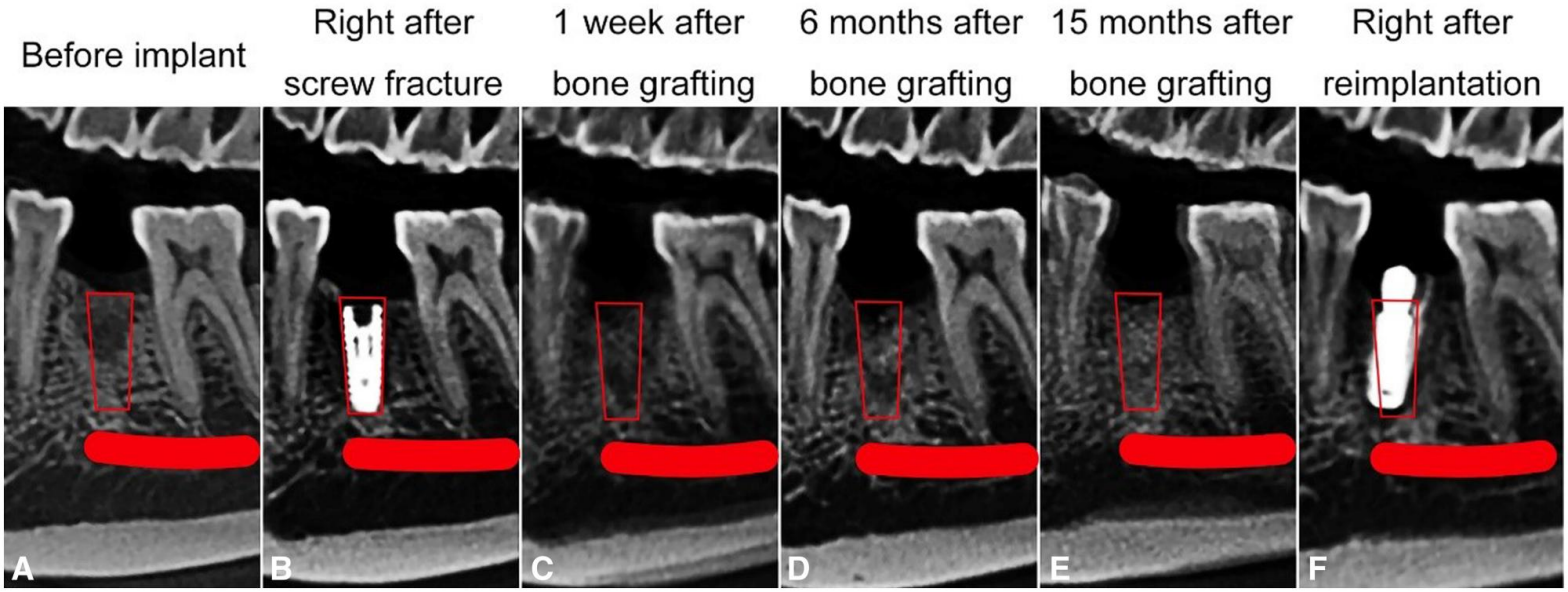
Sagittal CBCT sections at different time points: (A) before implant, (B) immediately after screw fracture, (C) 1 week after bone grafting, (D) 6 months after bone grafting, (E) 15 months after bone grafting and (F) immediately after reimplantation. The red box represents the bone defect, and the red line indicates the mandibular nerve canal.
